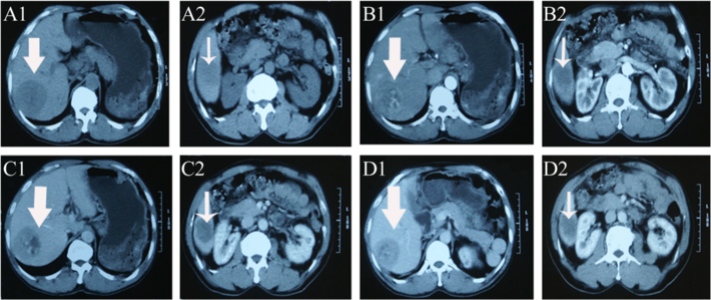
Computed tomography (CT) imaging of the patient with a double hepatic cancer of hepatocellular carcinoma and intrahepatic cholangiocarcinoma. Abdominal unenhanced CT showed two separate low-density tumors, in SVII (A1, *large arrow*) and SVI (A2, *small arrow*) of the liver. On contrast-enhanced dynamic CT, the SVII (B1, *large arrow*) tumor was enhanced, and the SVI (B2, *small arrow*) tumor showed peripheral enhancement during the arterial phase. Followed by wash-out during the portal venous phase (C1, C2) and the delayed phase (D1, D2): during the portal venous phase, the SVII (C1, *large arrow*) and SVI (C2, *small arrow*) tumors showed negative enhancement, and the density of SVII tumor rapidly decreased. During the delayed phase, the density of SVII (D1, *large arrow*) tumor further decreased, and the SVI (D2, *small arrow*) tumor showed slightly centripetal enhancement
